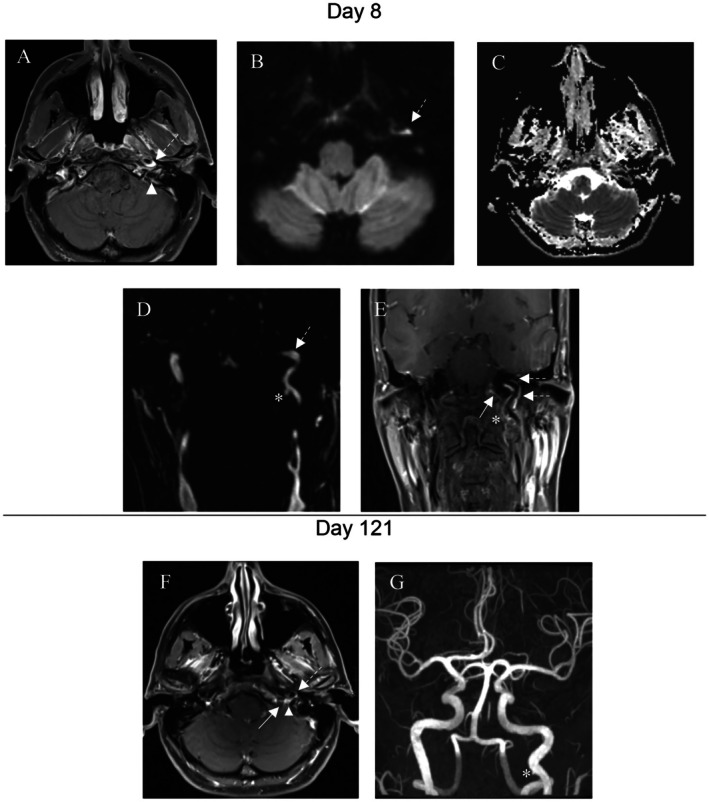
Radiological assessment at presentation and follow‐up. (A–E) Scull base MRI 8 days after symptom onset. (A) Axial imaging with T1 weighted TSE sequence and contrast media depicting jugular foramen (arrowhead) and dissection (dashed arrow). (B, C) DWI sequence showing diffusion restriction of dissection (dashed arrow), with corresponding ADC sequence. (D, E) Angiography in coronary plane with contrast media and visualization of dissection (dashed arrow) and pseudoaneurysm (asterisk) in close proximity to hypoglossal canal (white arrow). (F, G) Follow up after 121 days showing almost complete involution of both findings in axial scull base MRI. (F) T1‐weighted TSE with contrast media and (G) TOF magnet angiography. ADC, apparent diffusion coefficient; DWI, diffusion weighted imaging; MRI, magnet resonance imaging; TOF, time‐of‐flight; TSE, turbo‐spin‐echo.
